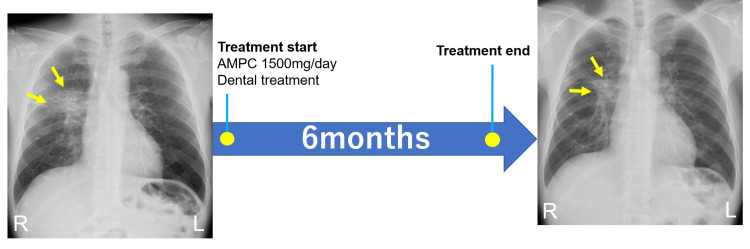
Treatment time course. The final diagnosis of pulmonary actinomycosis was followed by oral administration of AMPC 1,500 mg/day (two tablets, three  times daily), concurrent dental treatments. After six months, there was no hemoptysis, and although scar formation was observed on the chest X-ray, improvement in the lung consolidation and significant reduction of the right lung lesion were noted, indicating a favorable clinical outcome. R: right L: left, AMPC: amoxicillin.
